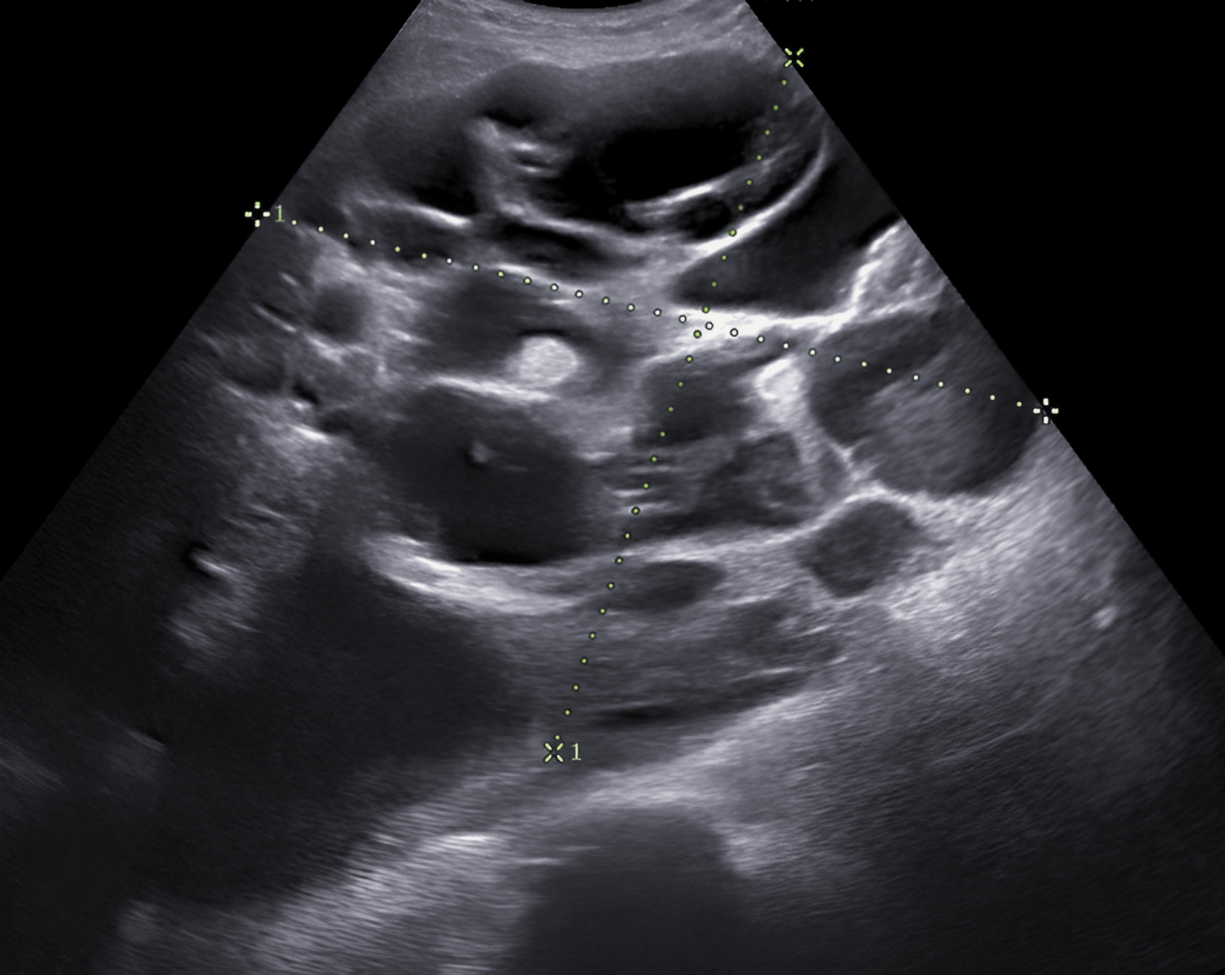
Transabdominal pelvic ultrasound It shows a markedly enlarged right ovary measuring 13.74 × 12.43 cm, with multiple large thin-walled cysts.
